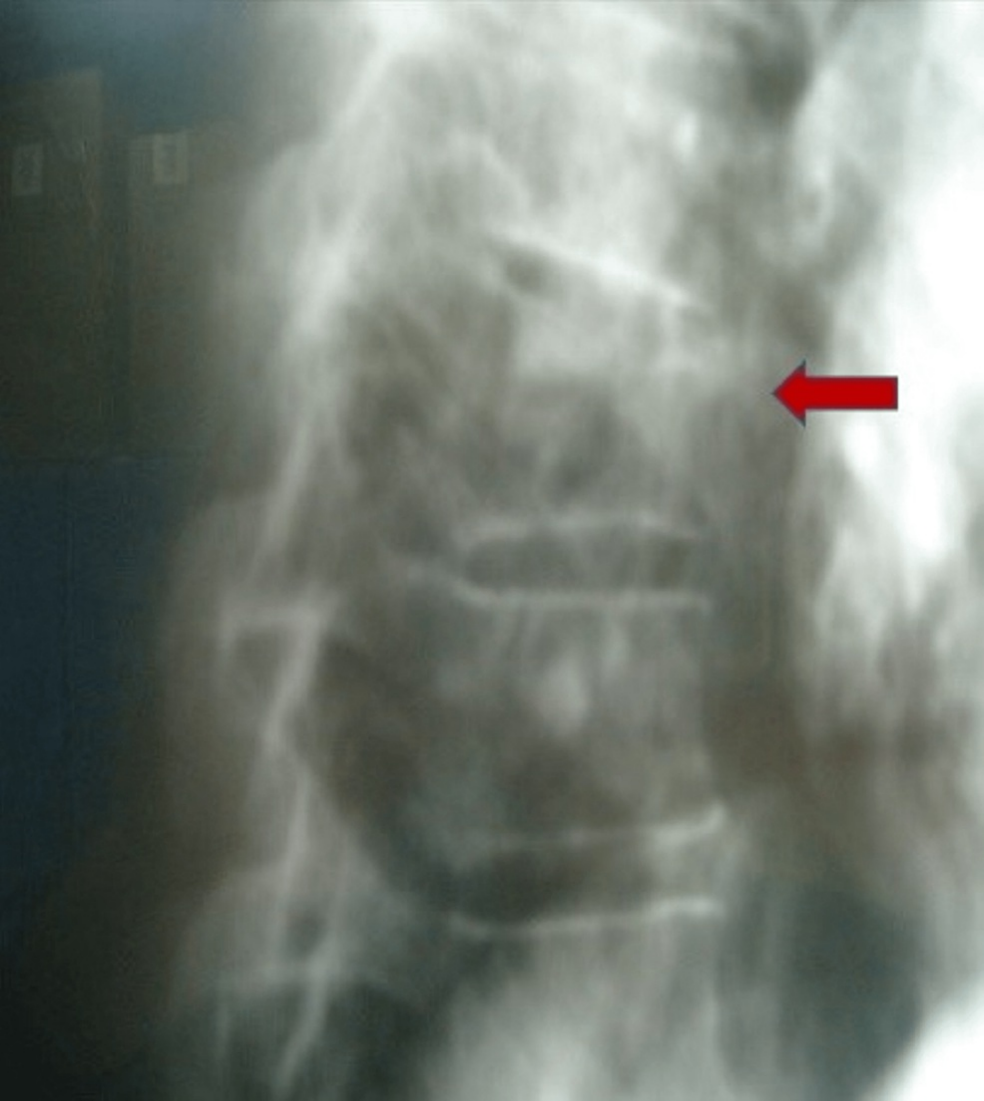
Lateral X-ray of the thoracic spine. The red arrow is the showing the T8 fracture.
